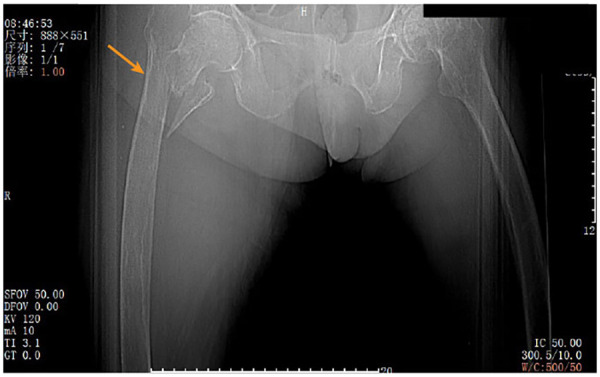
Digital radiograph (DR) revealing the right comminuted intertrochanteric fracture performed on August 15, 2024.
